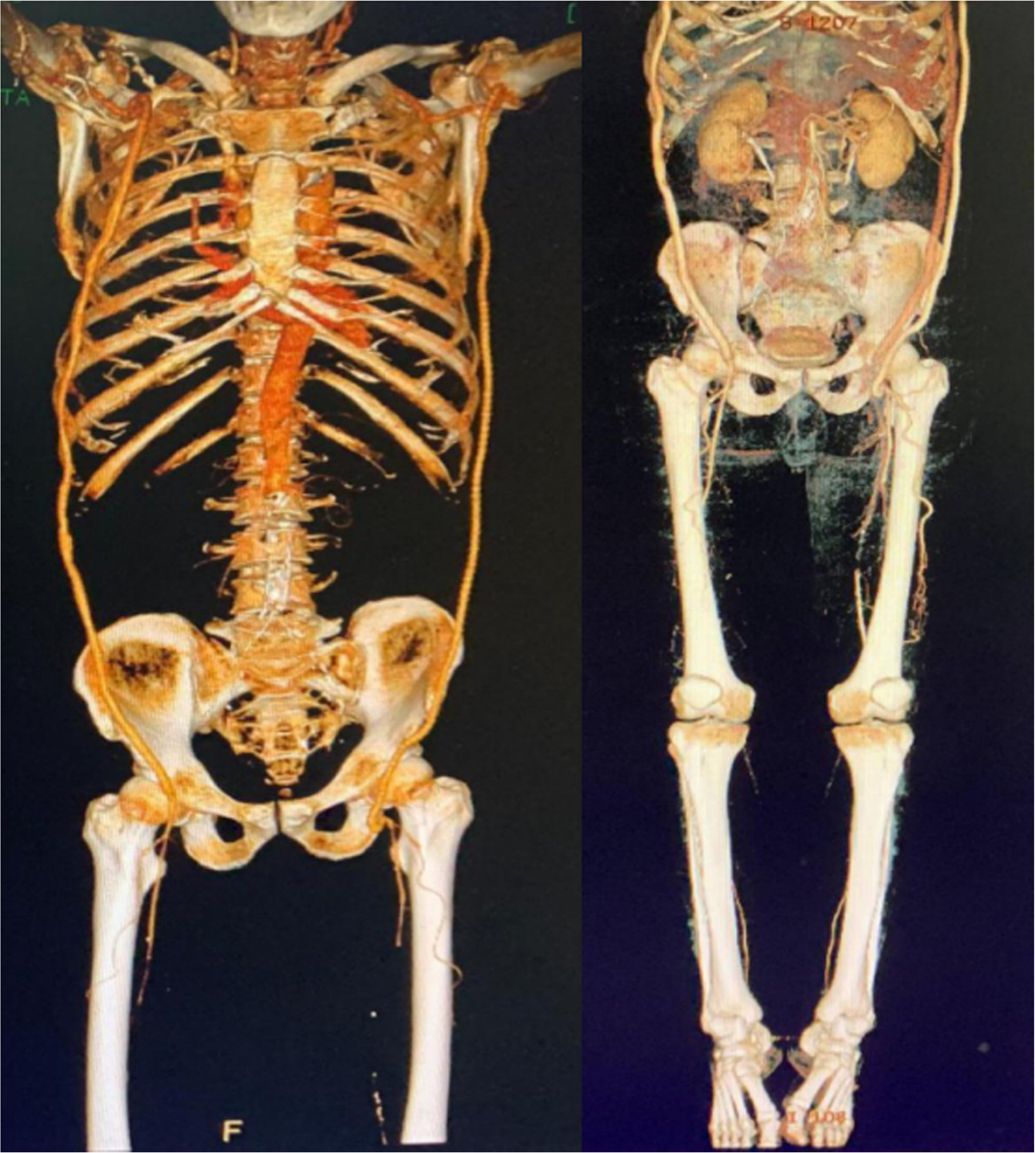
Postoperative CTA examination showing that the left LAx-PF bypass is unobstructed and that the previously occluded left axillary-femoral bypass vessel is visible. The right axillofemoral bypass is unobstructed
